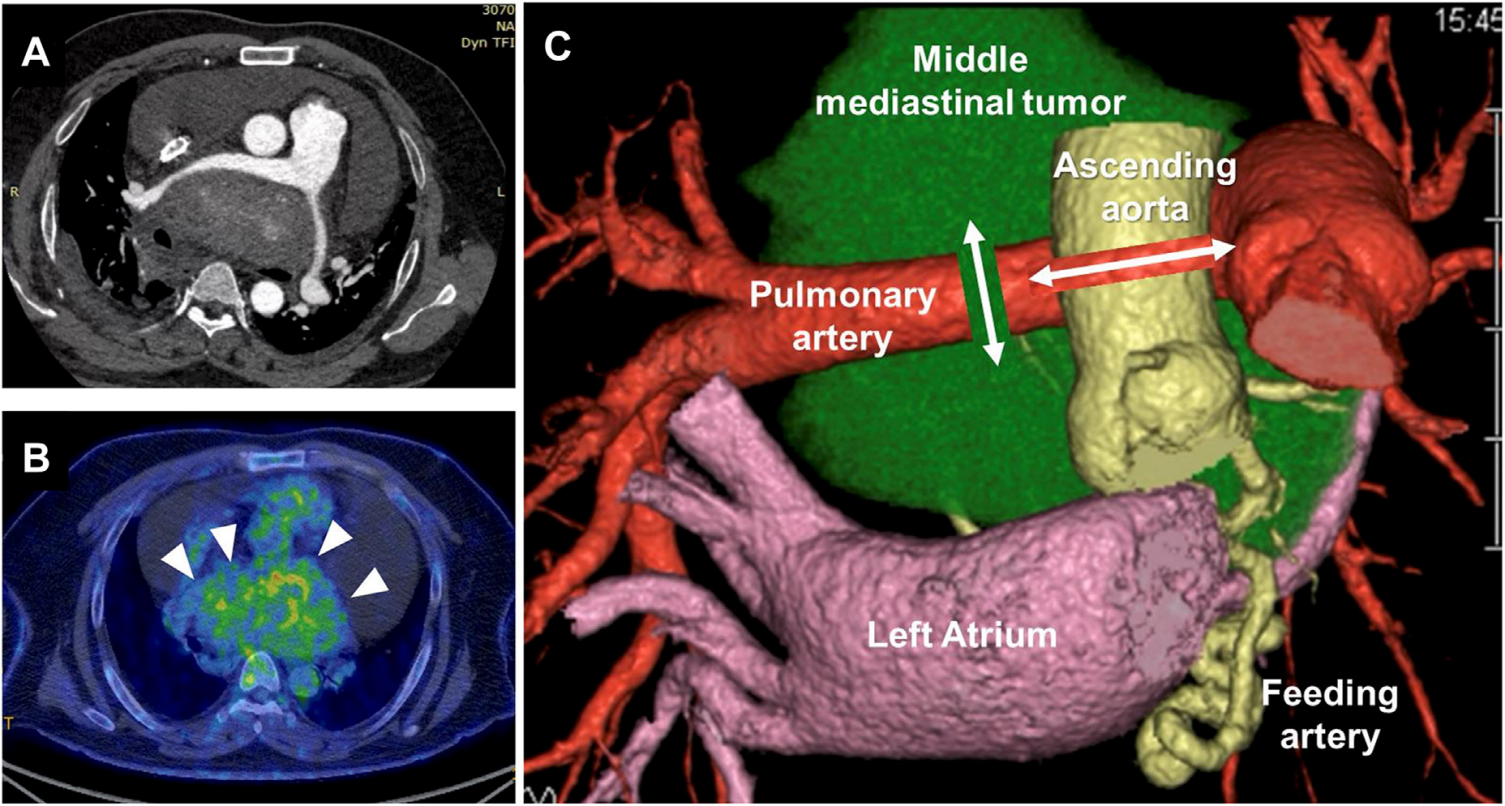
(A) Computed tomography (CT) shows a giant middle mediastinal tumor. (B) Positron emission tomography-CT shows moderate fluorodeoxyglucose uptake (arrowheads). (C) Three-dimensional CT shows feeders from the left coronary artery and transecting sites of the ascending aorta and right pulmonary artery.
